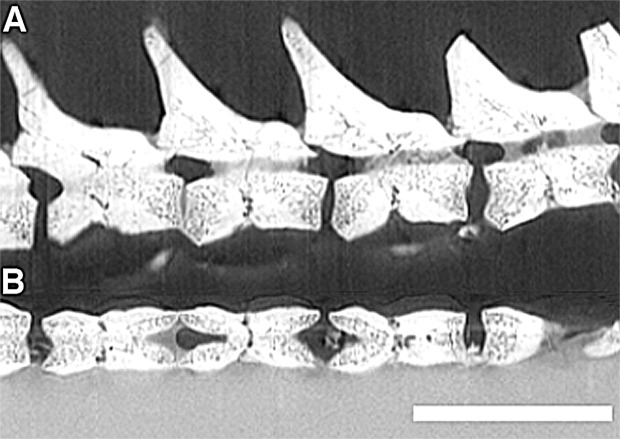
Micro computed tomography data of *Stereosternum*, PIMUZ A III 591, showing virtual sections through part of the caudal series in (A), sagittal and (B), transverse planes. Scale bar equals 4 mm.
